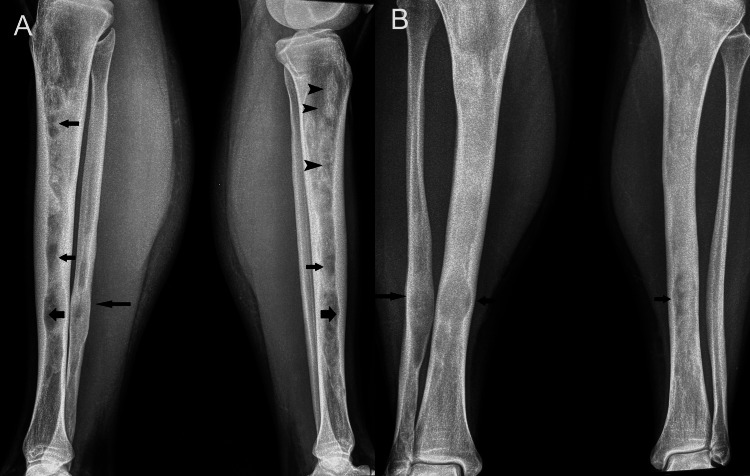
Oblique and lateral (A) and anteroposterior (B) radiographs of the tibia and fibula on both sides show extensive alterations of polyostotic fibrous dysplasia featuring multiloculated, mixed radiolucent (arrows) and radiodense (arrowheads) lesions involving the entire shaft of the bones. Associated endosteal scalloping (thick arrows) and cortical thinning are seen. Note focal bone expansion mostly in the fibula (long arrow).
